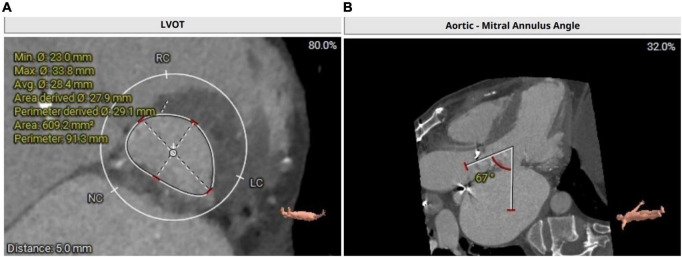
(A) Calculation of left ventricular outflow tract with an area of 609.2 mm^2^. (B) CT depicting a steep aorto-mitral annulus angle with 67°.
